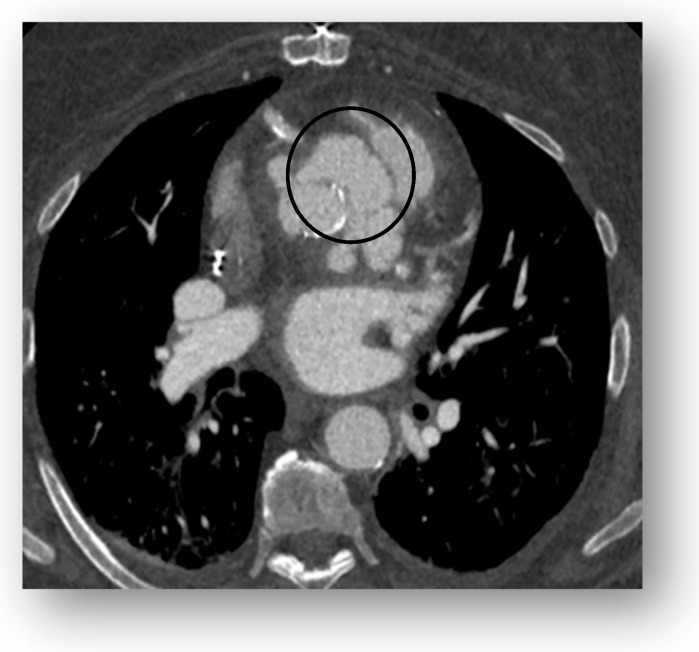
This picture shows an aortic root endocarditis complicated by a pseudoaneurysm (circle) suspected on TTE, later confirmed by CT scan. This is an original, de-identified clinical image from our institution. Patient consent for publication was obtained.
